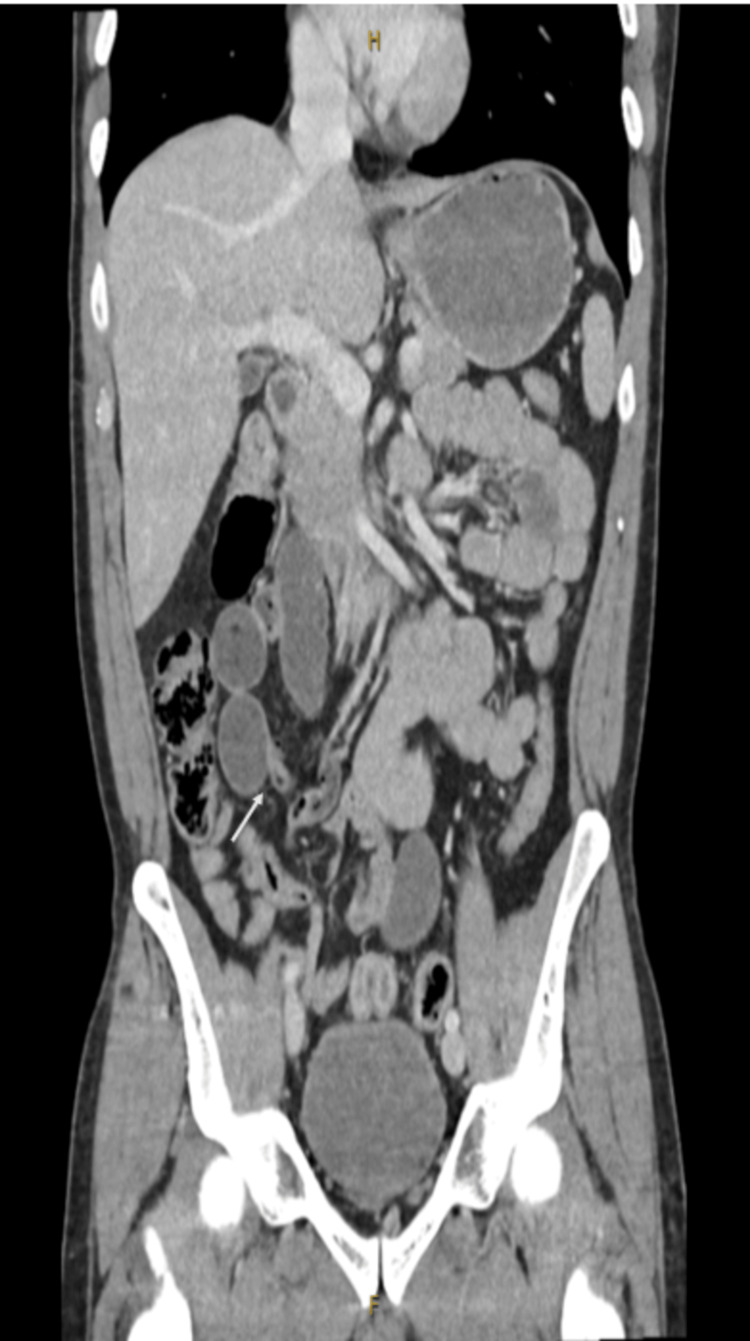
Coronal view of the CT scan revealing small bowel loops measuring up to 3 cm and collapsed distal small bowel. The arrow pointing towards the transition point.
